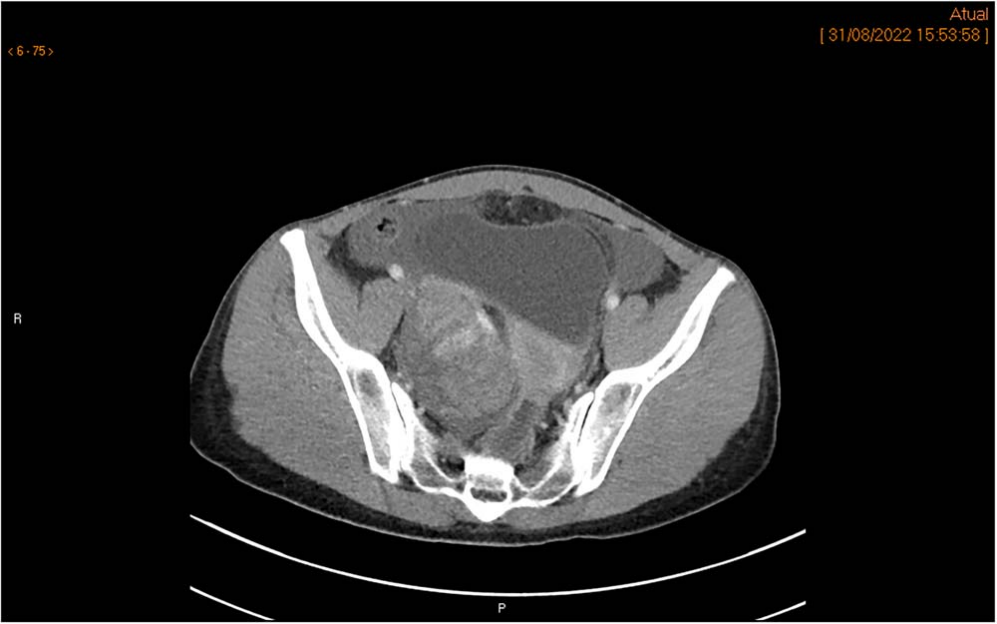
CT scan demonstrates a right ovarian mixed lesion measuring 80 × 67 × 57 mm, with solid, contrast-enhancing components, ascites, and prevesical fat stranding nodule, with no other categoric signs of carcinomatosis.
